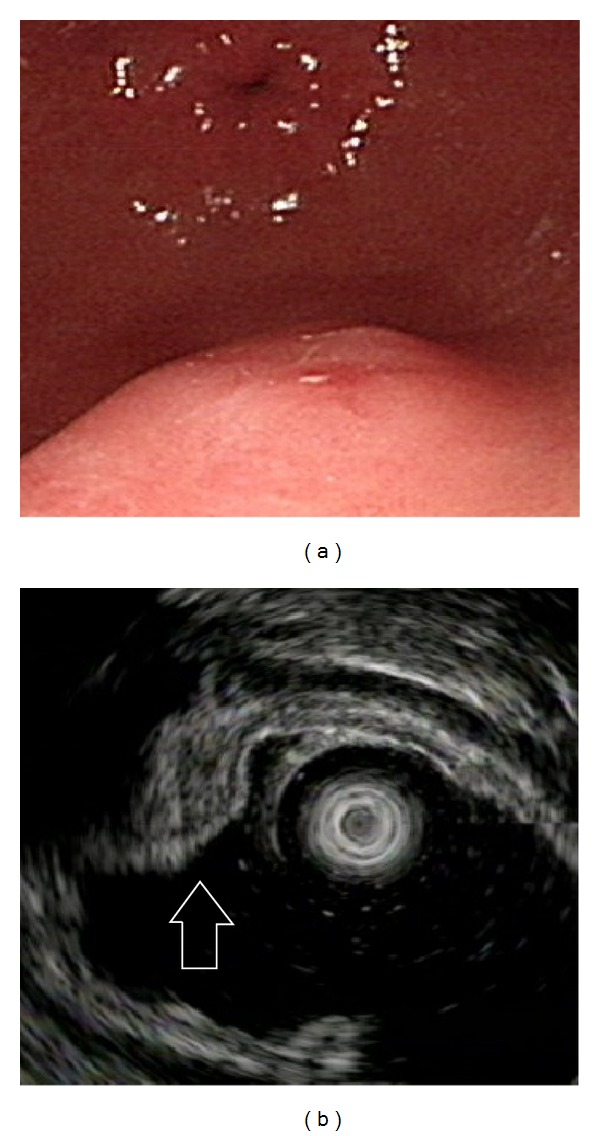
Ectopic pancreas originates from the second and third sonographic layers of the gastric wall. (a) A subepithelial tumor with intact but uneven surface was identified in the antrum. (b) Endoscopic ultrasonographic image obtained with a 12 MHz catheter probe. The tumor exhibits heterogeneous, mixed echoic echogenicity and indistinct margins, involving the second and third sonographic layers of the gastric wall (arrow).
